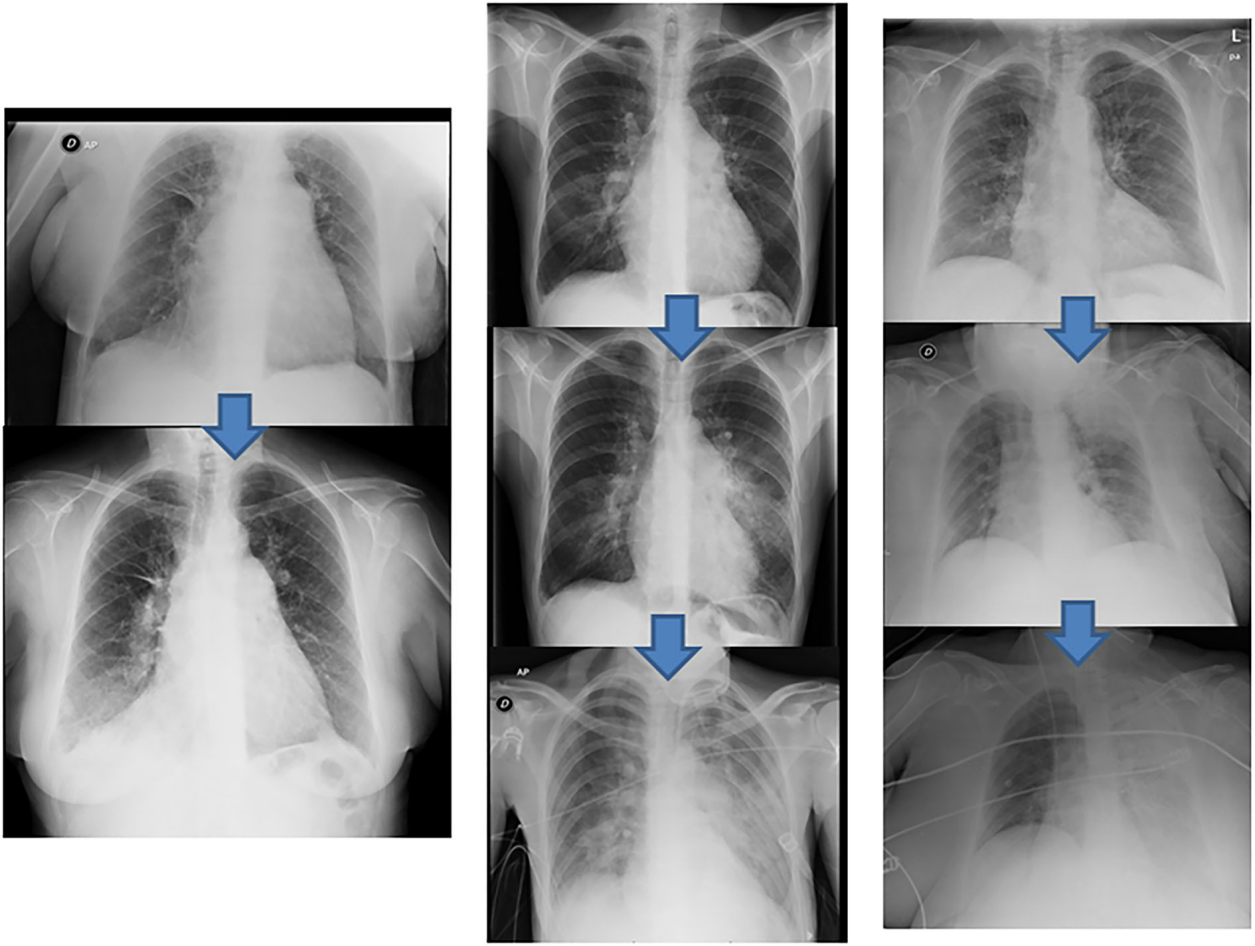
Radiologic pulmonary injury expression. Examples of different degrees of reperfusion edema according to their radiological expression. (Left) grade 3 reperfusion edema, infiltration in the right lower lobe (above pre-BPA baseline chest radiograph). (Center) grade 4 reperfusion edema, infiltration in the lingula extending to the contralateral lung (above pre-BPA baseline chest radiograph). (Right) grade 5 reperfusion edema, initial infiltration in the left upper lobe that eventually spreads to the entire left lung (above pre-BPA baseline chest radiograph). BPA, balloon pulmonary angioplasty.
